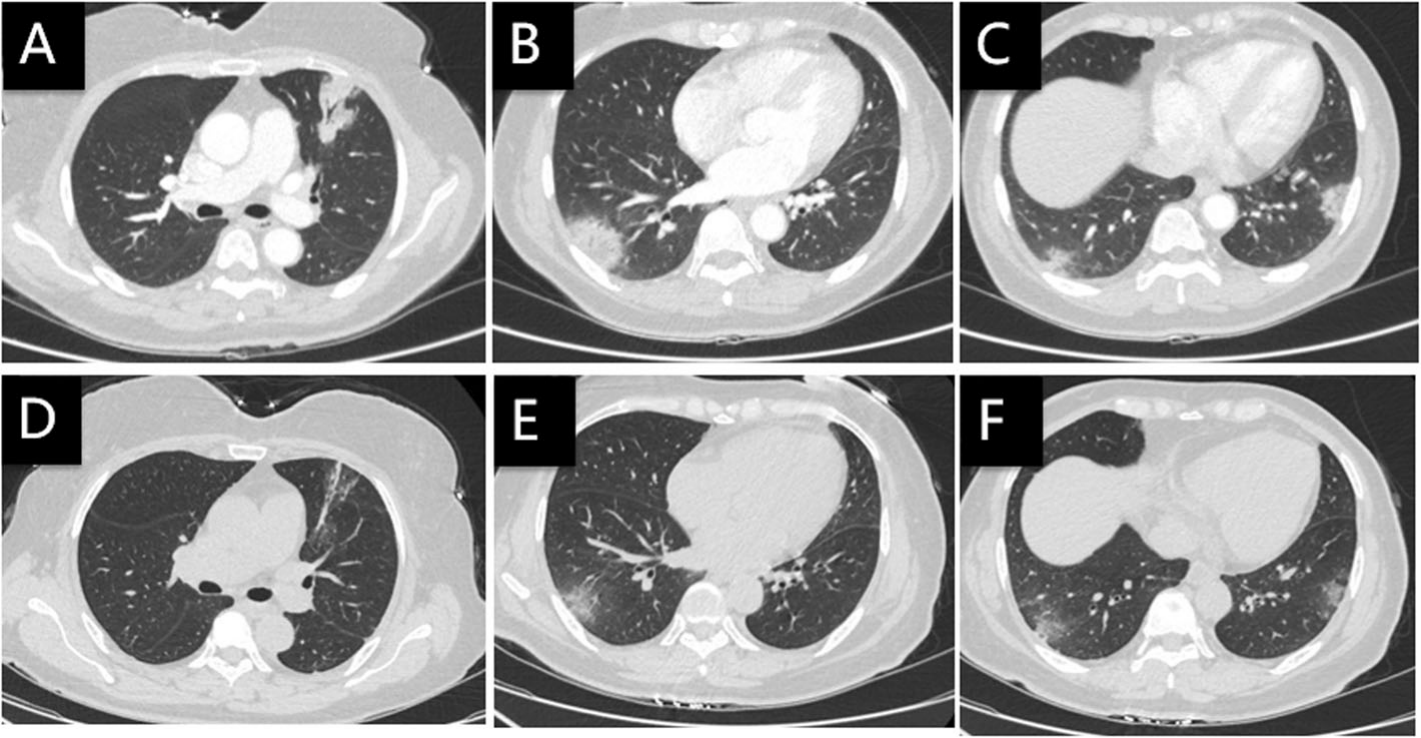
Chest CT of case 5: a, b, c On the day of admission, d, e, f Eight days after treatment with moxifloxacin
